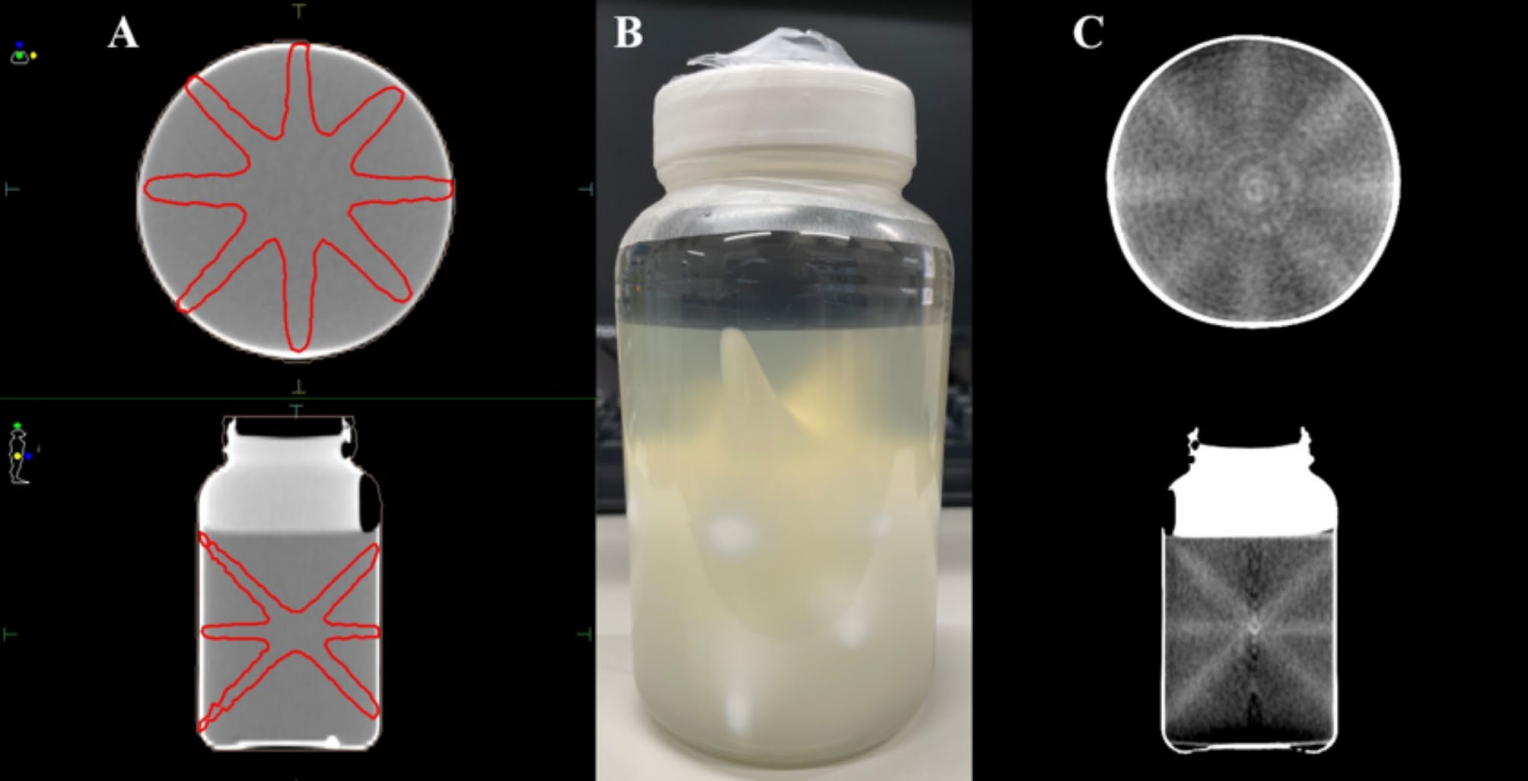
Three-dimensional starshot (3D-SS) treatment planning (A), irradiated gel dosimeter (B), and cone-beam computed tomography (CT) images of the post-irradiated gel dosimeter (C) used in this study. Red lines in panel A indicate the 20 Gy isodose line.
